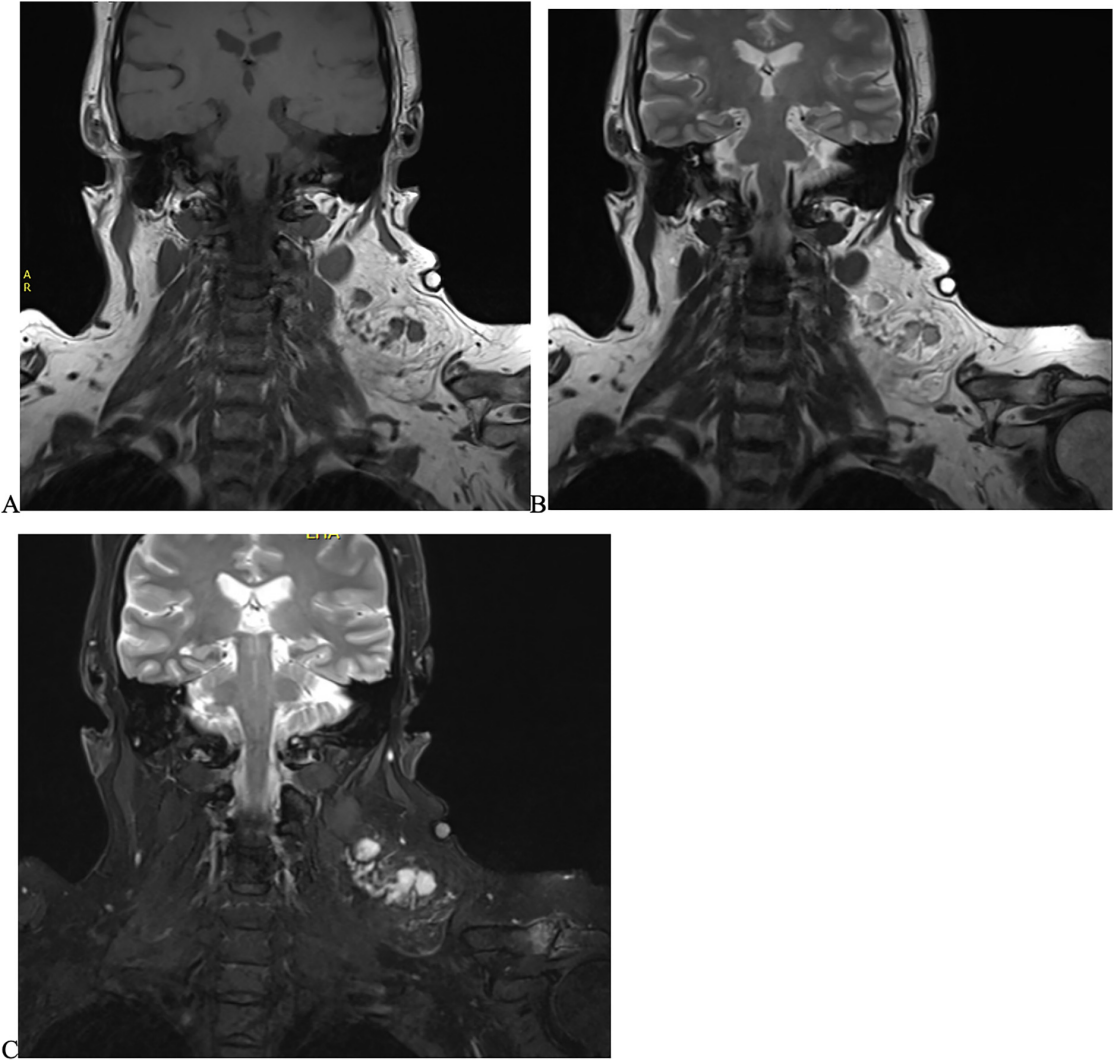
Imaging findings from the patient. Magnetic resonance imaging (MRI) findings show a complex lesion 7×5×6.5 cm in the left posterior triangle of the neck, with solid enhancing components. A, Coronal T1 MRI shows intermediate T1 enhancement of the 6 central solid nodules. (B) Coronal T2 MRI shows high T2 signal enhancement. The lesion does fat saturate. (C) Coronal post-gadolinium (Gd) enhancement shows high Gd enhancement, the nodules have restricted diffusion.
